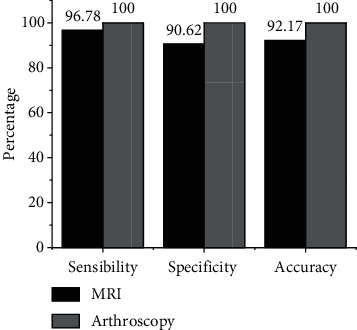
Contrast of MRI diagnosis performance of ACL injury.
